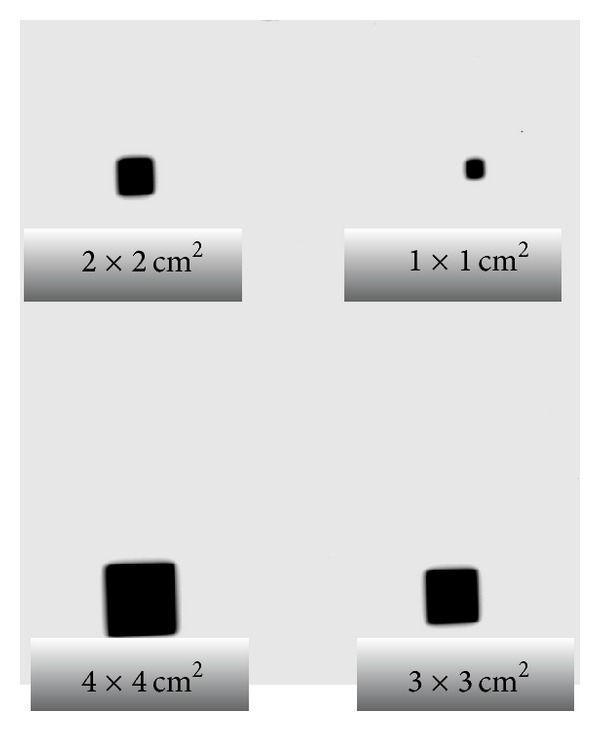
Radiographic film exposed to 6 MV for various fields.
